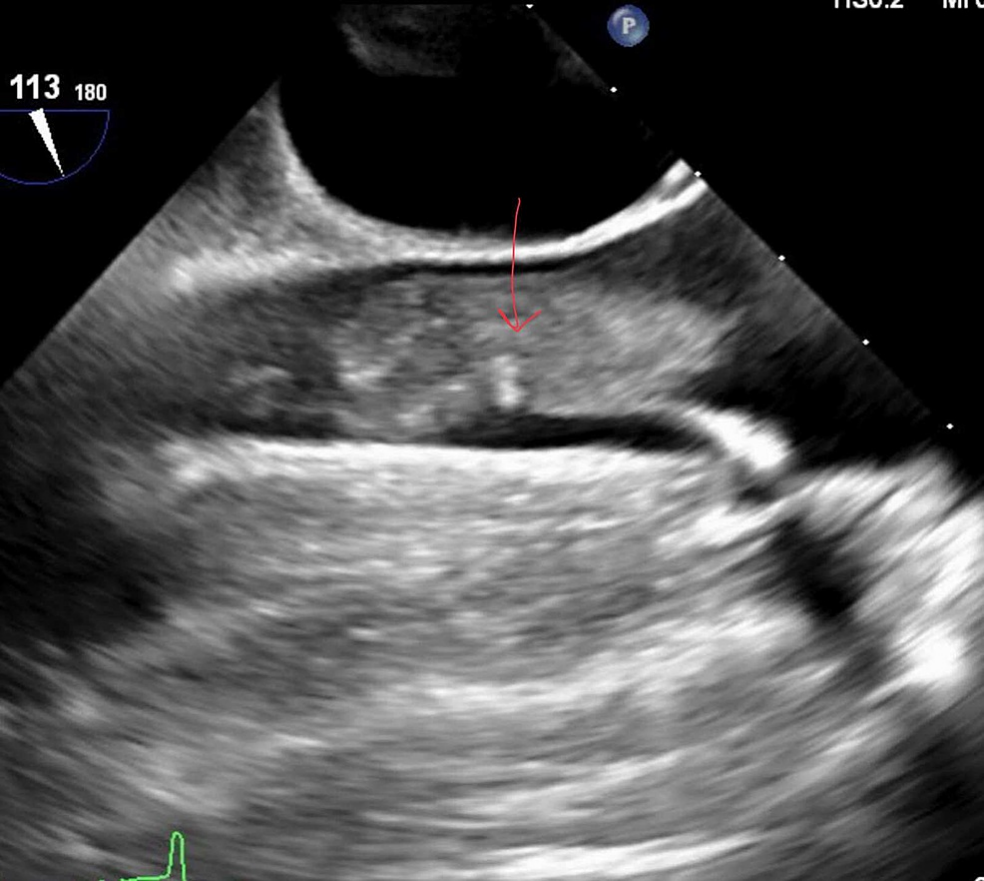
TEE indicates 2.7 × 1.0 cm vegetation in the superior vena cava TEE: transesophageal echocardiography
